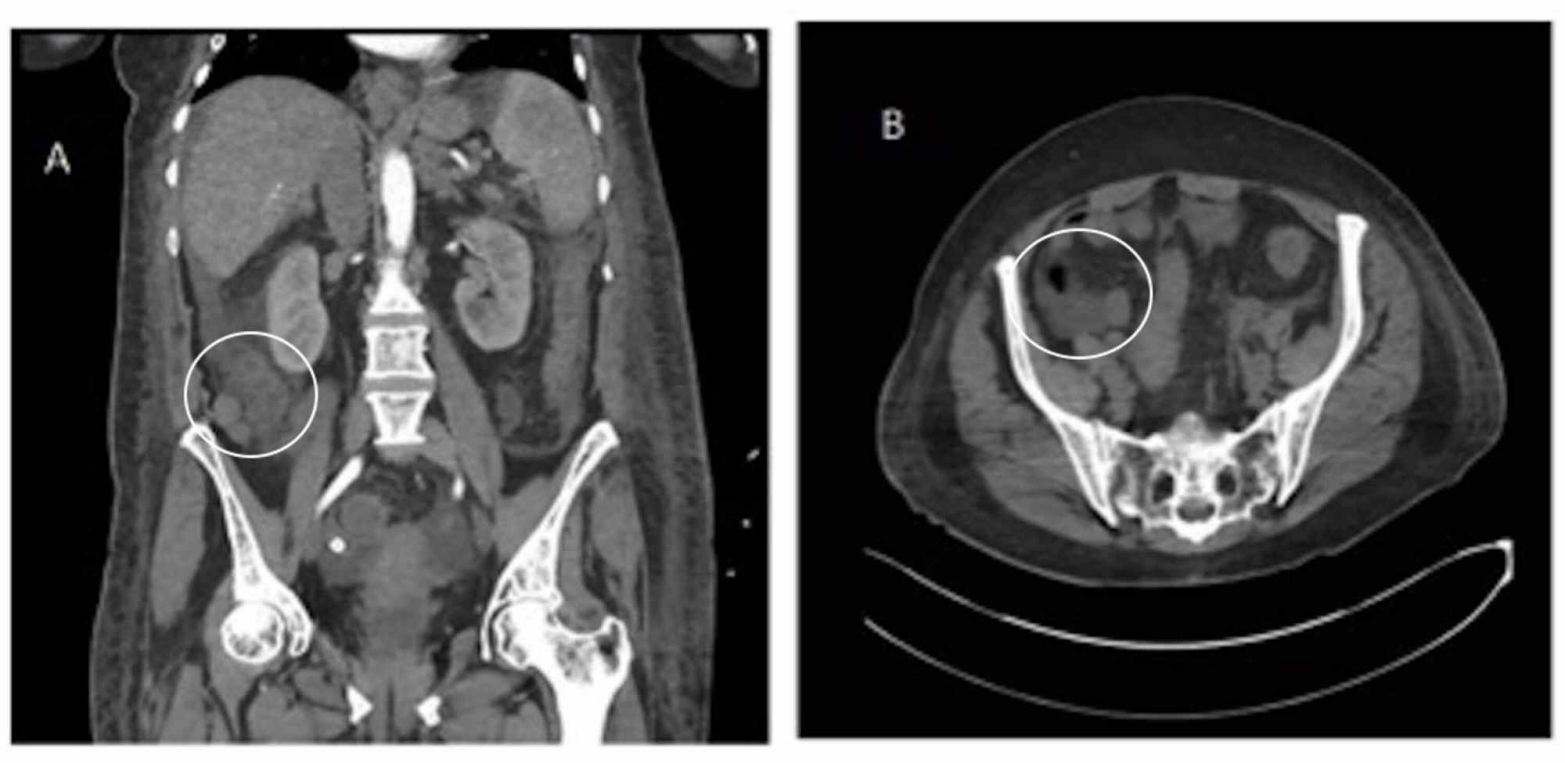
CT angiography in the venous phase. Second CT angiography shows no extravasation of contrast, indicating no active bleeding. Filling of the cecal varix (circled) is visualized in the venous phase of angiography. A: AP view. B: transverse view AP, anteroposterior
